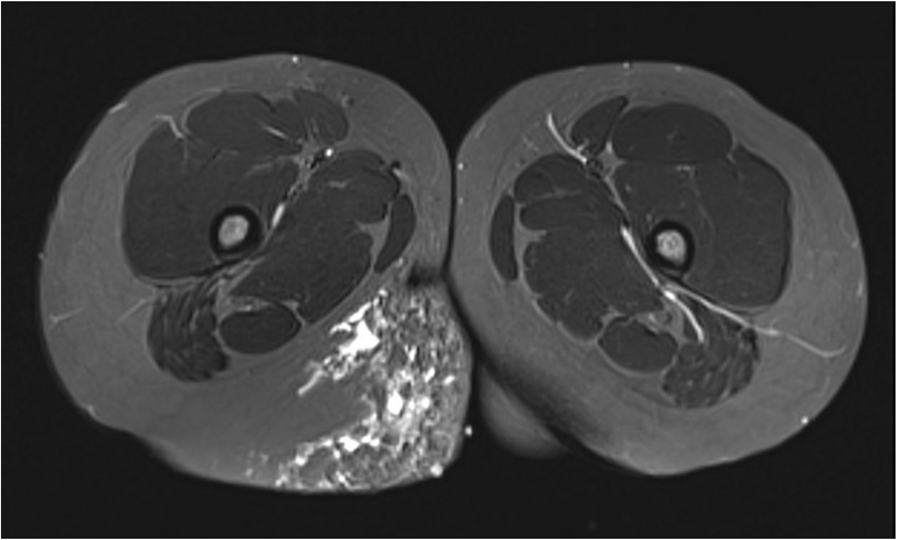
Axial T2 weighted MR image of a 9 year old male with a lymphatic malformation of the right buttock. The subcutaneous lymphatic cysts, which are of high T2 signal, are of varying sizes and there is additional fat present, contributing to the bulk of the lesion
